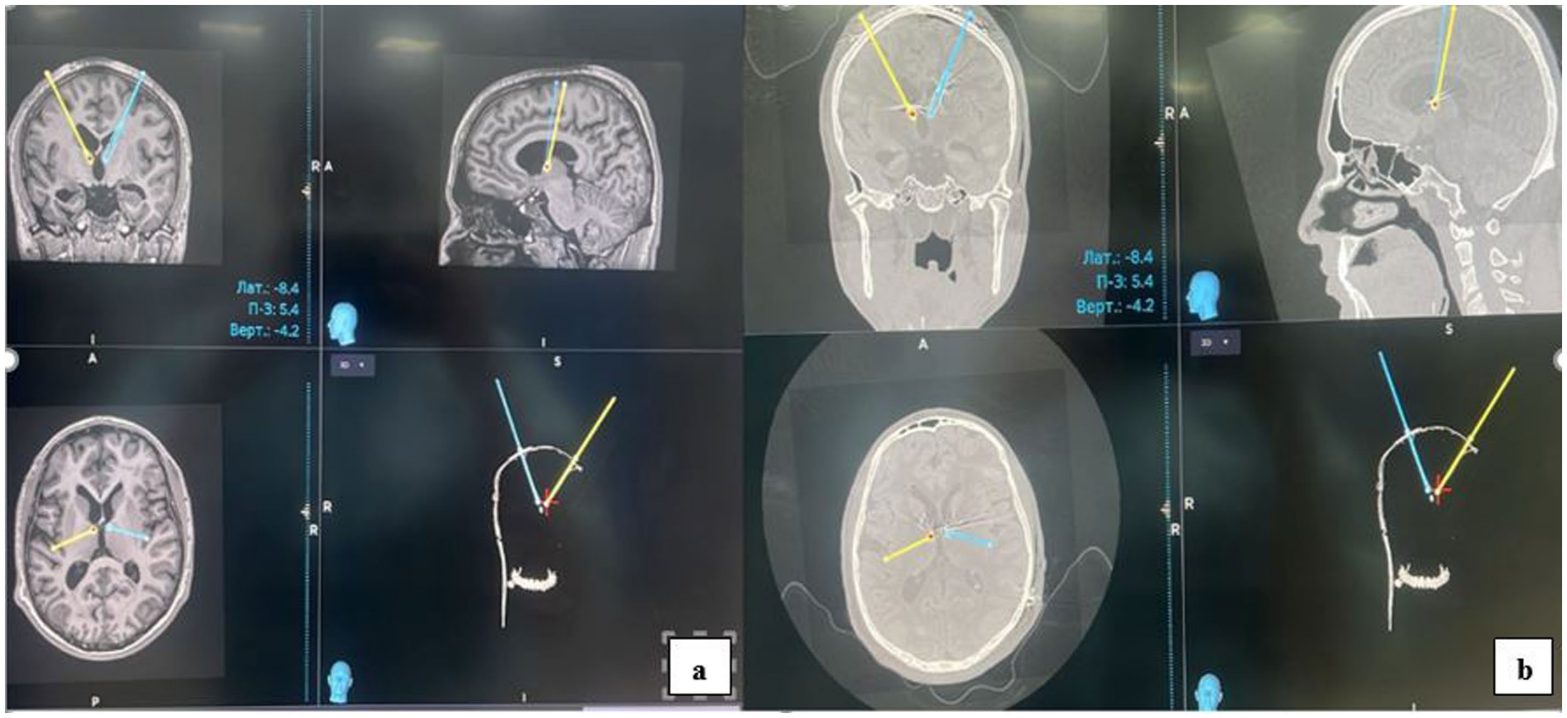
Postoperatively, electrode placement was confirmed by fusing postoperative CT scans (b) with the preoperative planning MRI (a).
